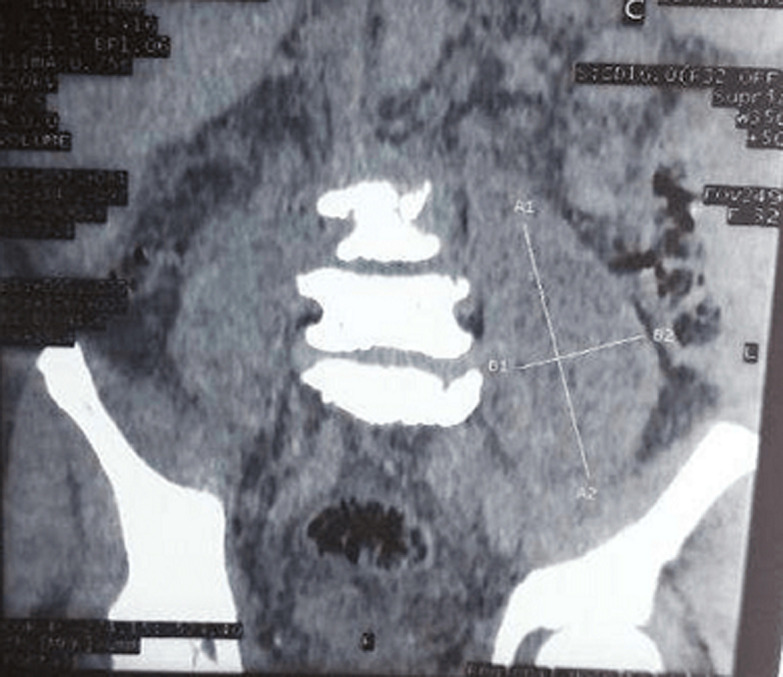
left psoas abscess on abdominal CT scan
